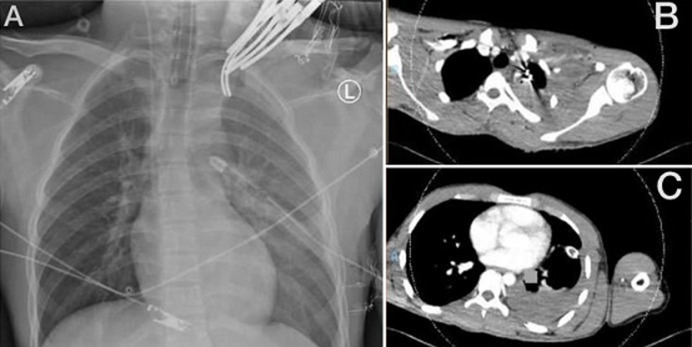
A) plain chest X-ray, anteroposterior view in the supine position at presentation; B) computed tomography scan of the lower neck/upper chest showing subclavian vessel injury; C) computed tomography scan of the chest showing left sided hemothorax
